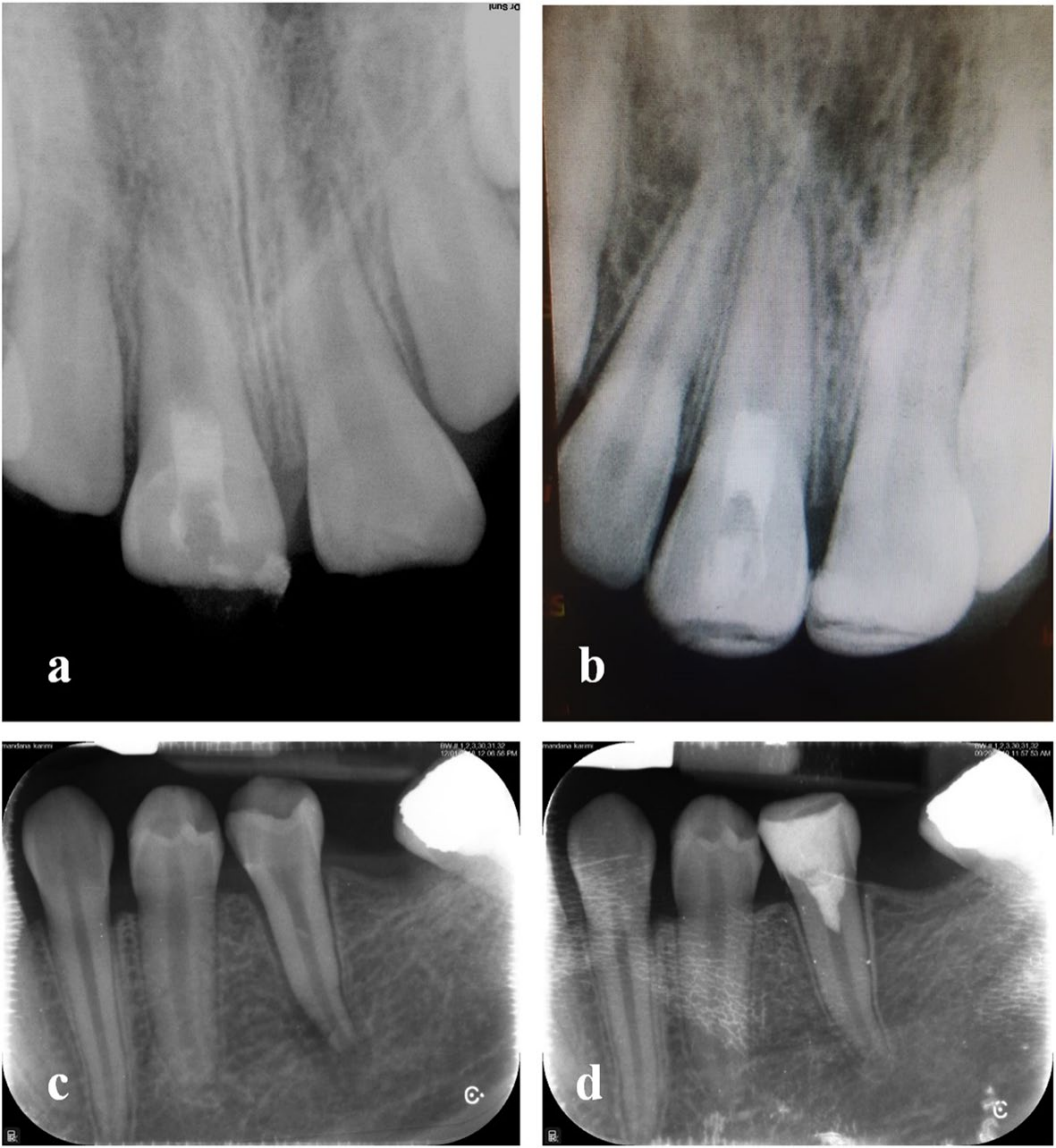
a Preoperative radiograph of tooth number 8. b At 24 month follow-up of tooth number 8. c Preoperative radiograph of tooth number 20. d At 18 month follow-up of tooth number 20
